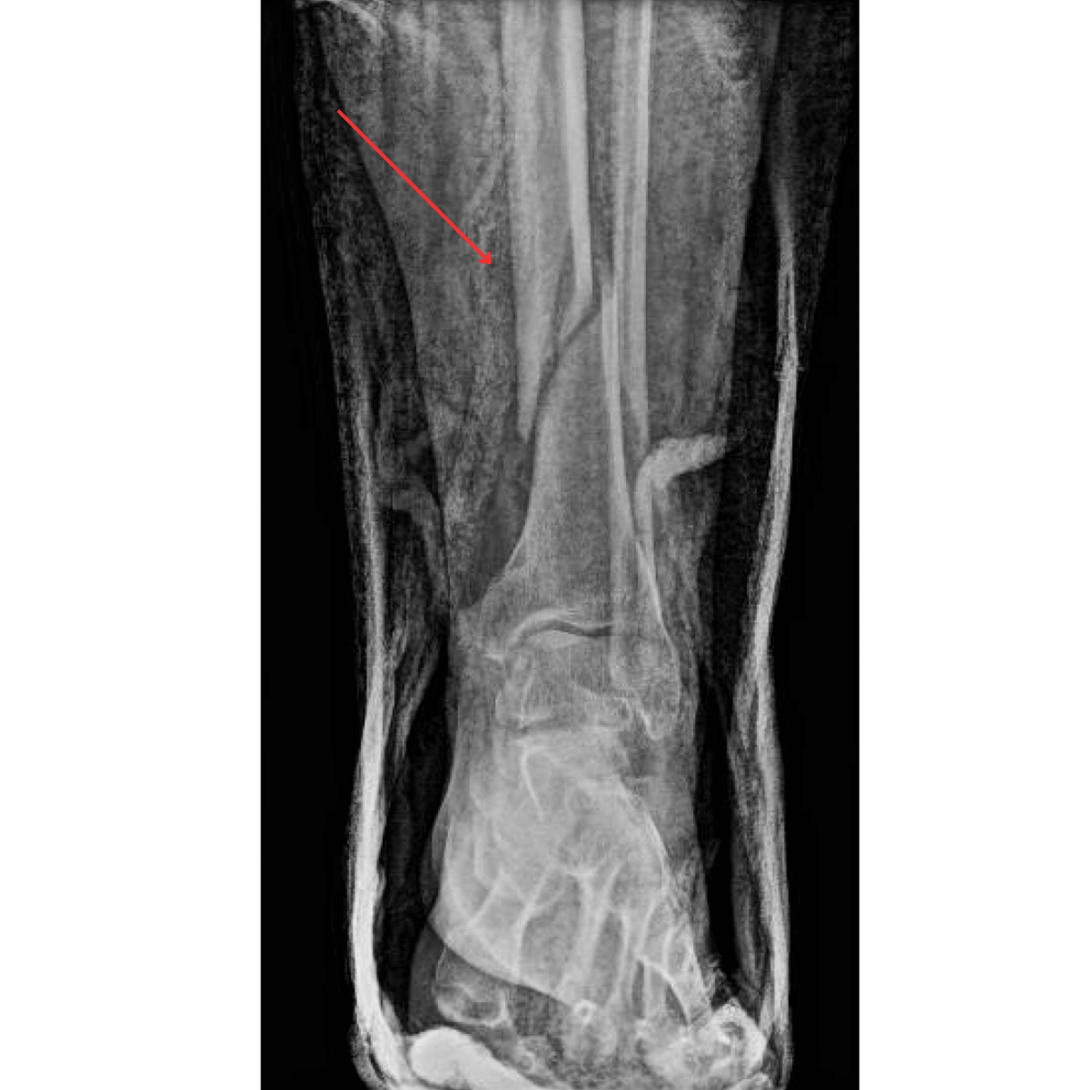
X-ray of the distal left tibia fracture
